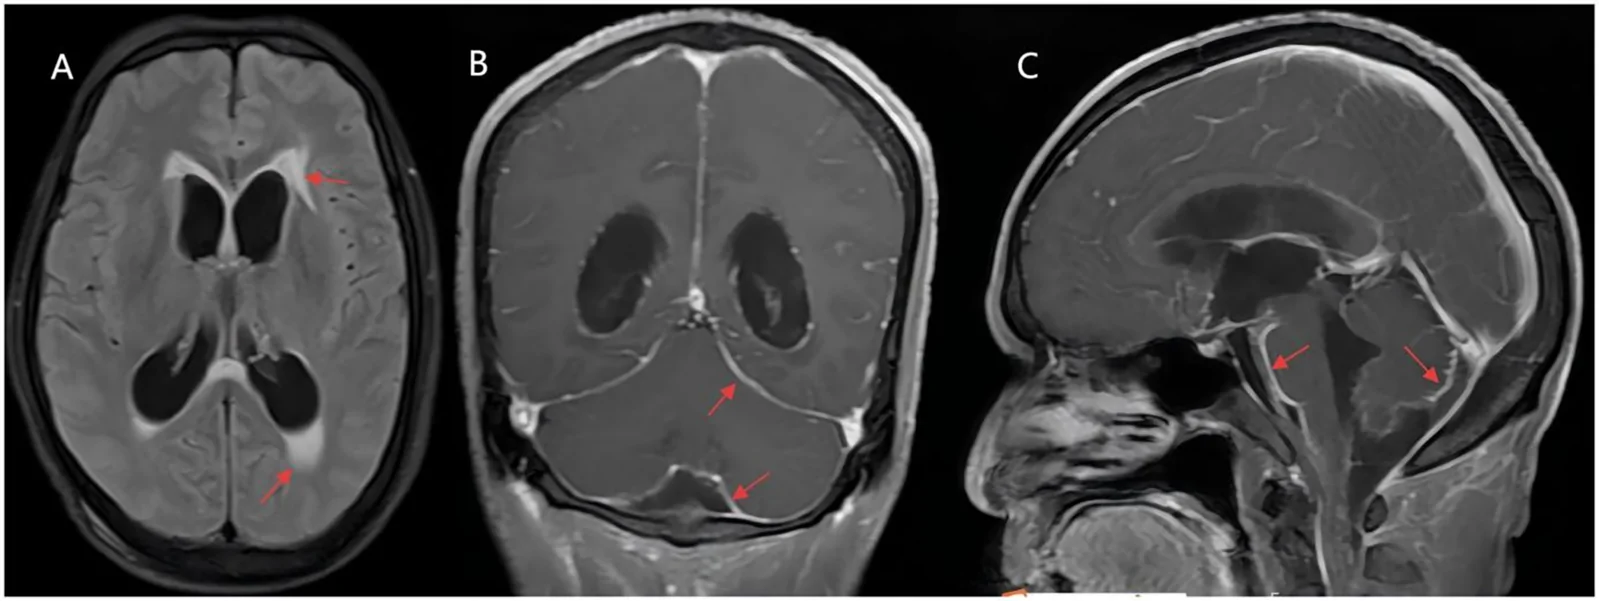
Cranial MRI findings on hospital days 4 and 5. (A) Axial T2-FLAIR image (day 4): ventricular dilatation and periventricular hyperintensities (red arrows) consistent with transependymal interstitial edema. (B) Coronal and (C) sagittal post-contrast T1-weighted images (day 5): diffuse leptomeningeal enhancement (red arrows) involving the basal cisterns, tentorium cerebelli, and brainstem surfaces.
